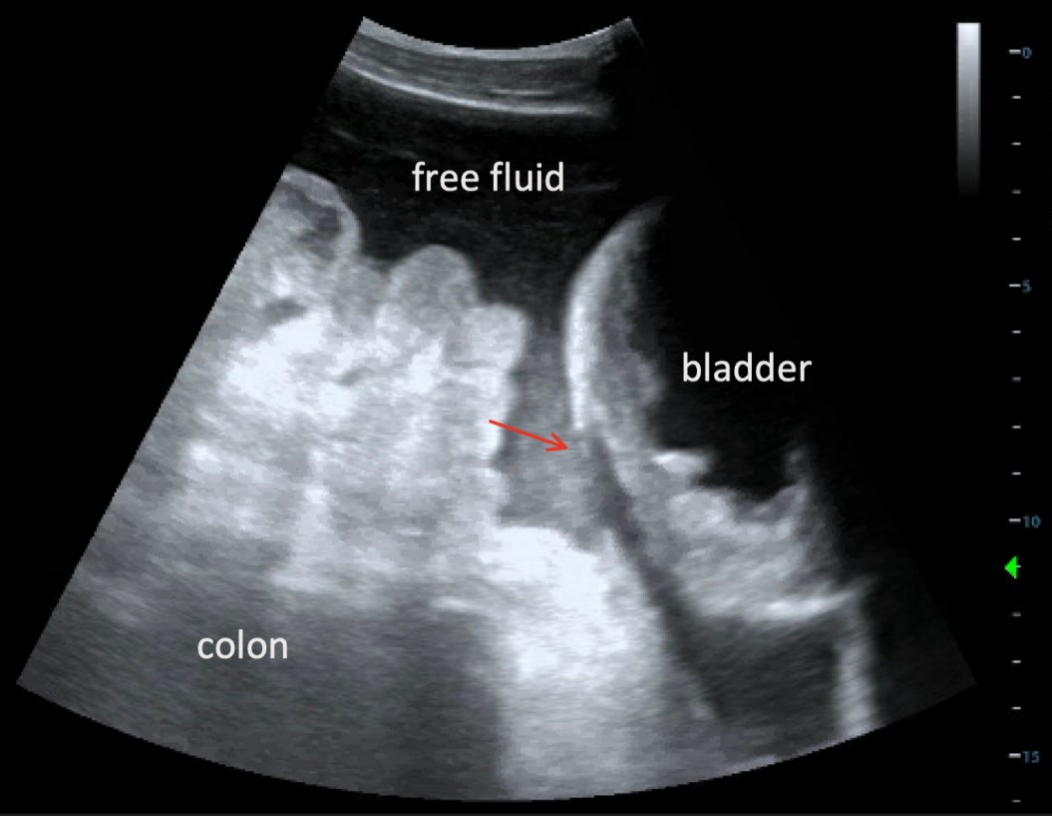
Point of care ultrasound in sagittal plane demonstrating the purported disruption in the hyperechoic bladder dome (red arrow).
